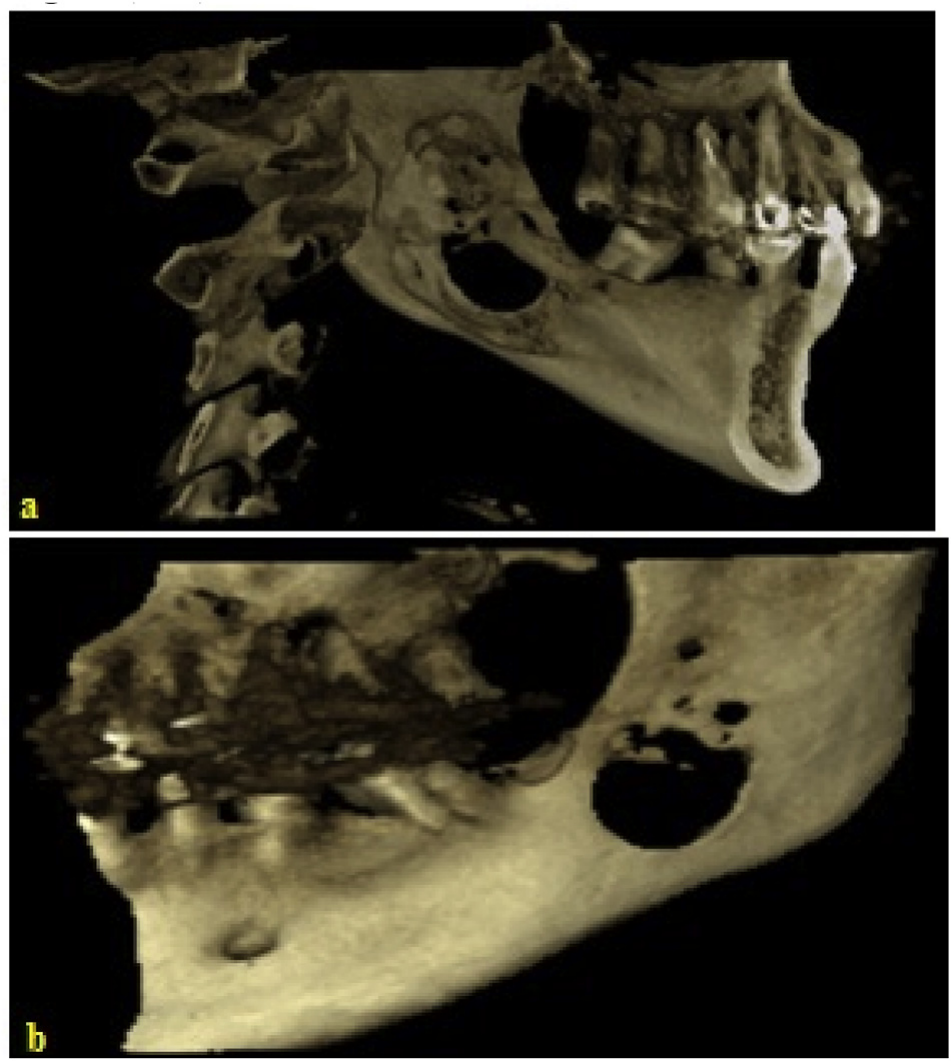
(a,b) 3D CT of the of the mandible.
